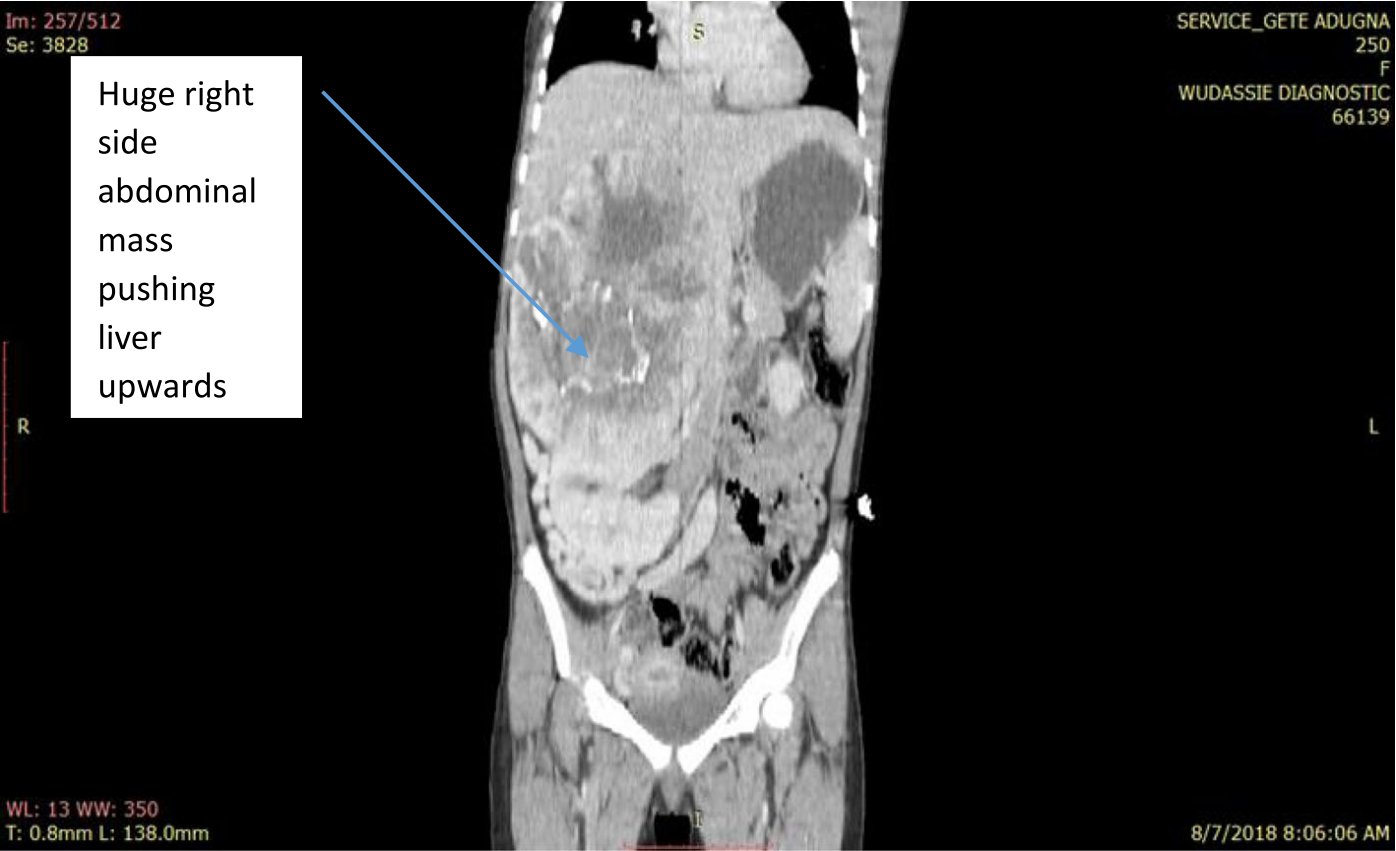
Computed tomography scan of solitary fibrous tumor of right adrenal in 13-year-old girl
